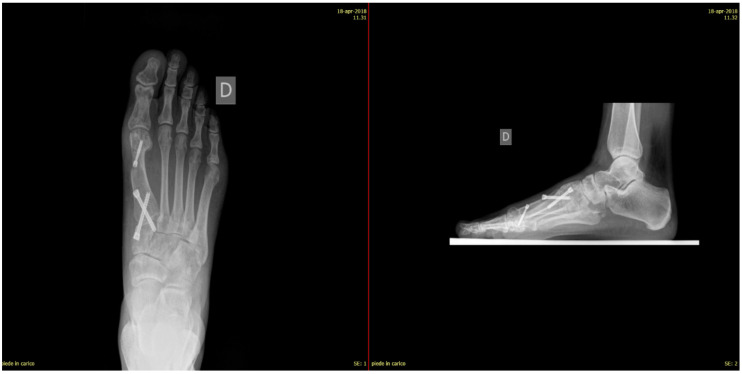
Three-month post-operative weight-bearing X-rays of the foot: dorsoplantar view (left) and lateral view (right).
